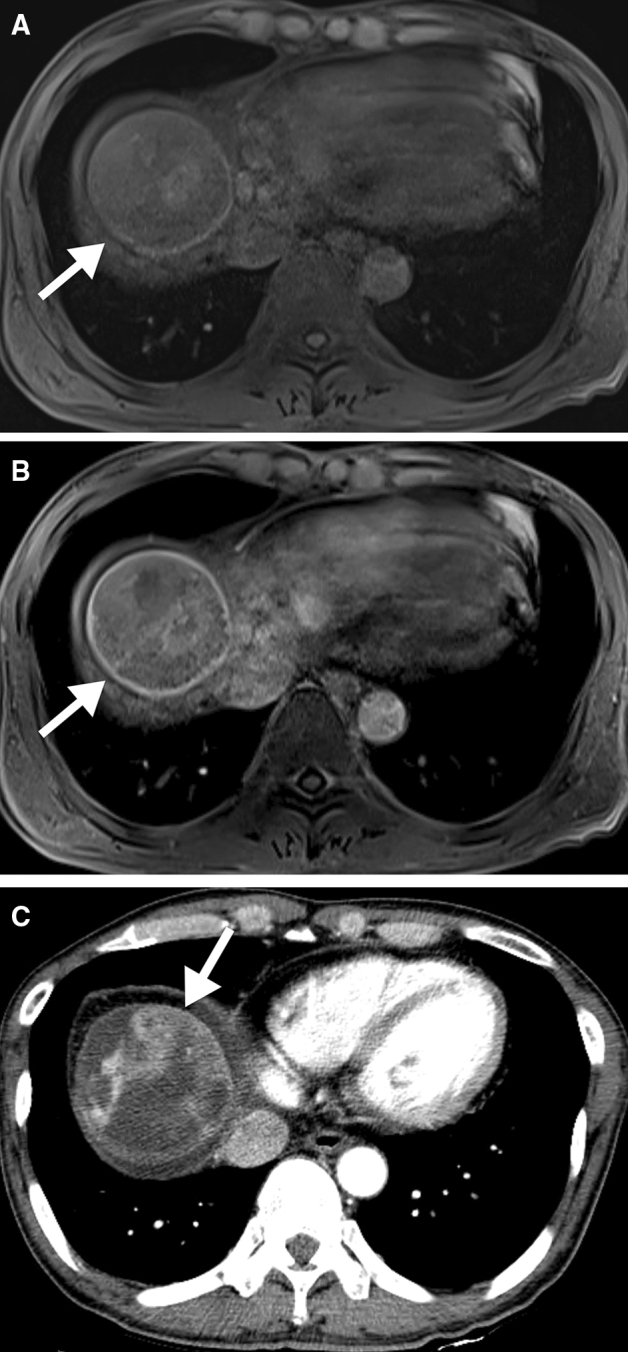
A 50-year-old man with hepatocellular carcinoma in hepatic dome (arrows). Gadoxetic acid-enhanced MR images show hypointensity in the precontrast scan (A) and heterogeneous signal intensity in the hepatobiliary phase (B). Residual tumor with arterial enhancement remains on a follow-up CT taken 27 days after DEB-TACE (C). Treatment response was determined to be a stable disease. *DEB-TACE* drug eluting bead transarterial chemoembolization, *CT* computed tomography, *MR* magnetic resonance.
